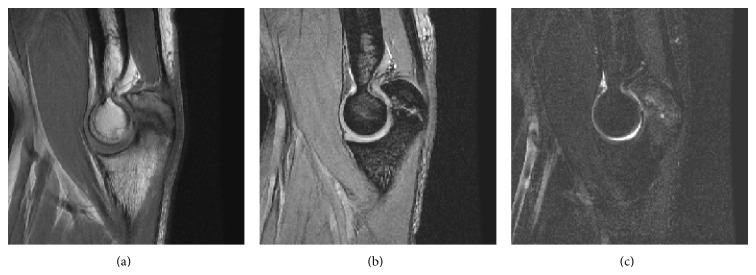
Sagittal T1-weighted (a) and T2-weighted (b) magnetic resonance (MR) images of the right elbow showing abnormal intensity in the olecranon physis. Sagittal T2-weighted MR image with fat saturation (c) of the right elbow showing high intensity in the intramedullary region.
